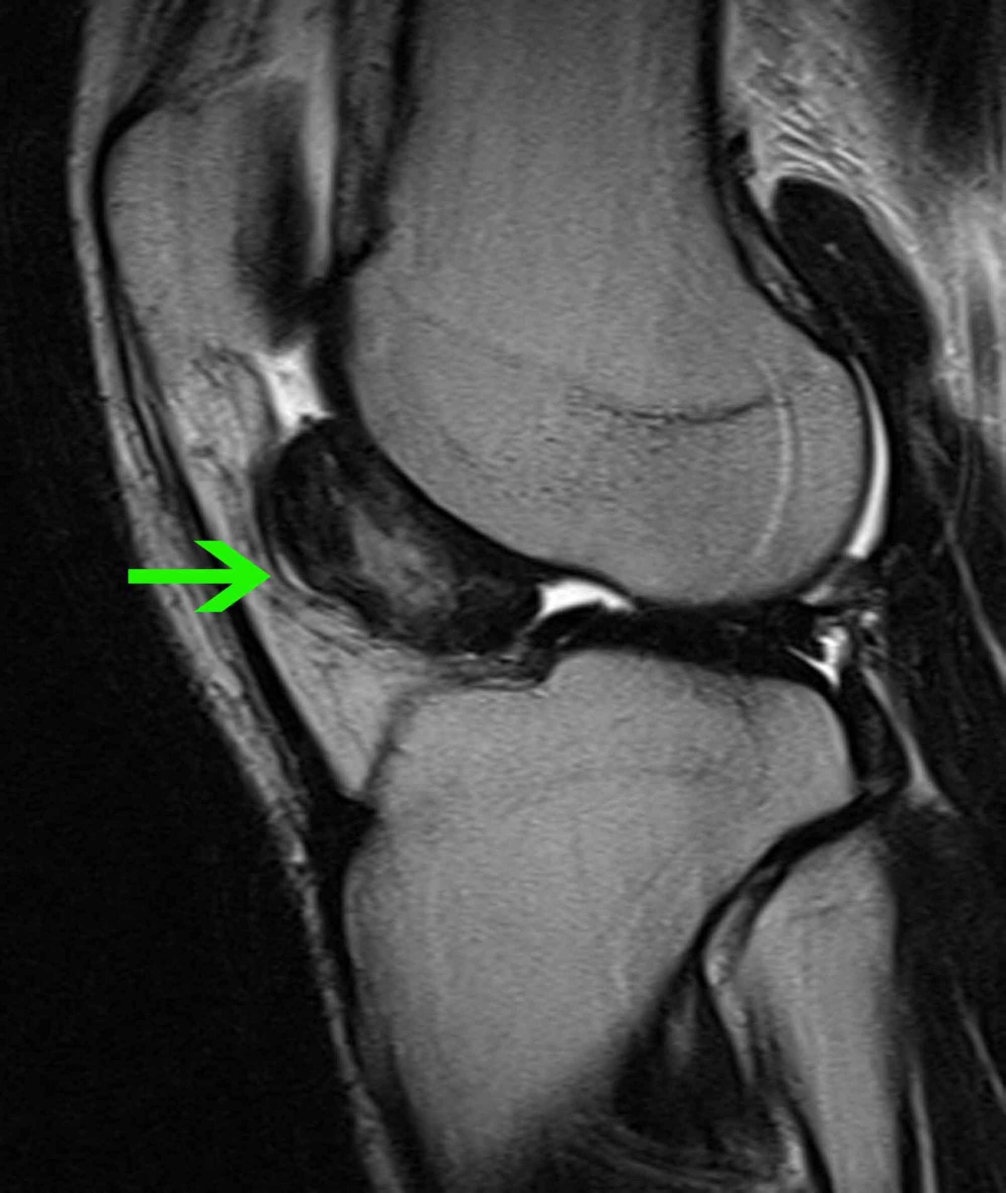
MRI of the left knee (sagittal plane) T2-weighted turbo spin-echo (TSE) sequence, sagittal plane, in which a heterogeneous signal mass can be seen posterior to the fat pad (green arrow).
